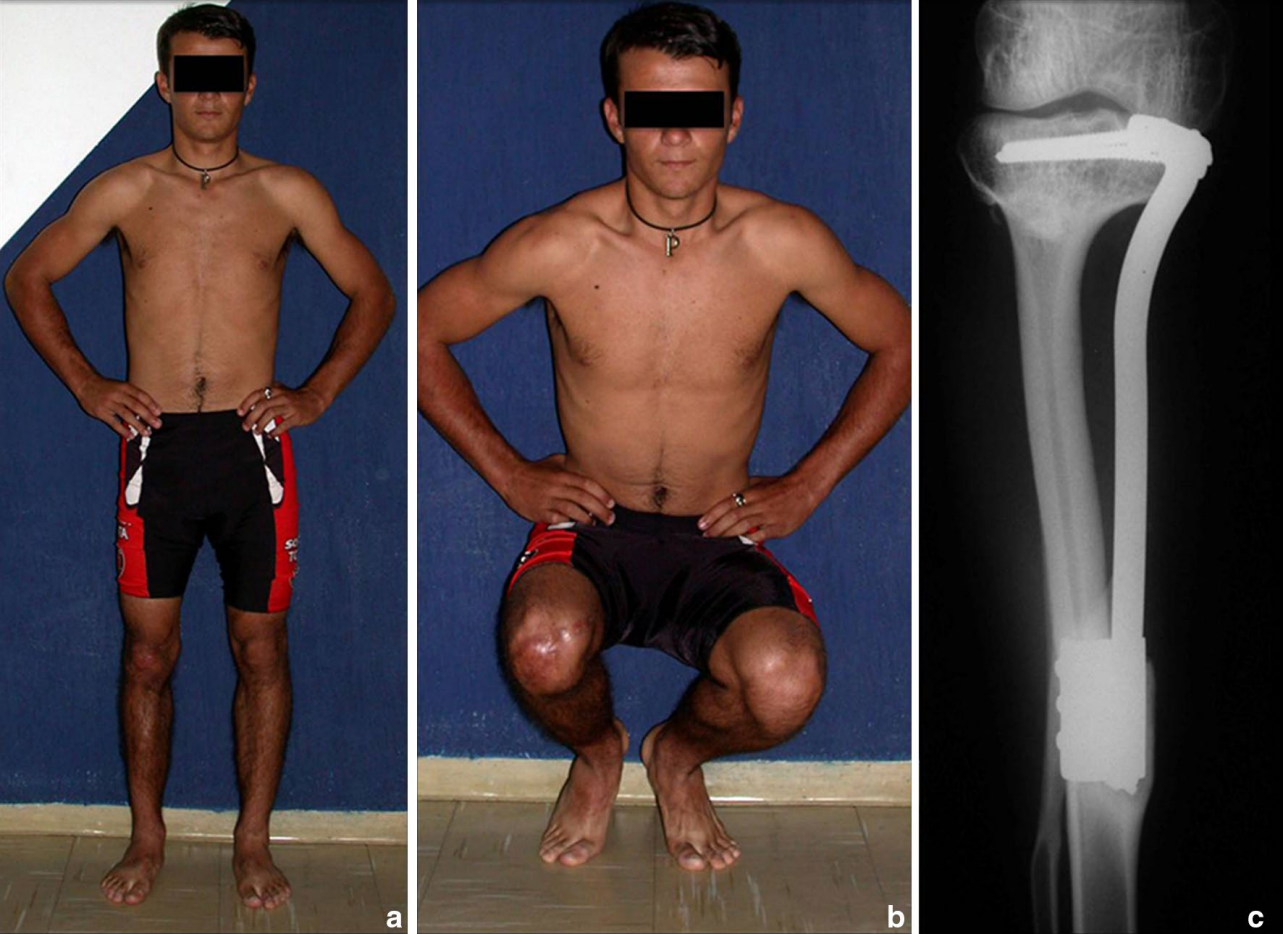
a patient 3 years and 7 months postoperatively, at full load, b 3 years, 7 months, bending under load and good function of the knee, and c radiograph 3 years, 7 months, fibula hypertrophied, already fully tibializated
